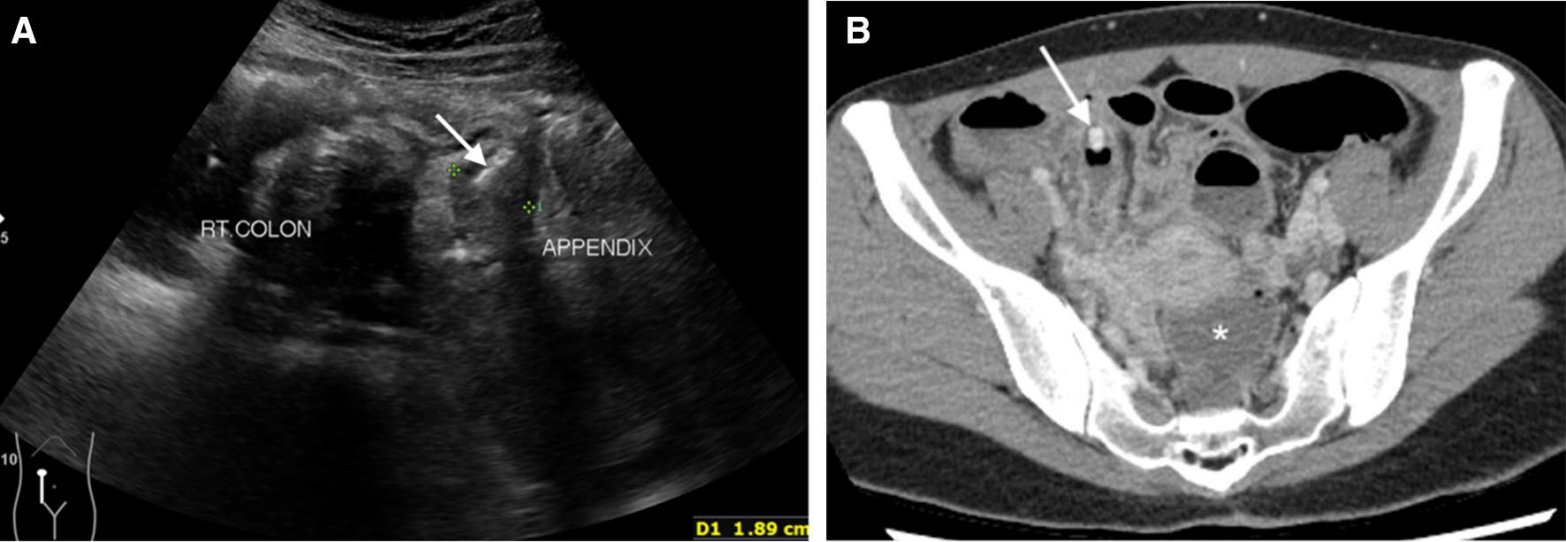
Intraluminal appendicolith. An ultrasound image a of a 35-year-old woman presenting with right lower abdominal pain for 1 day, elevated white blood cell counts (23,790 cells/mm^3^) and neutrophilia (93.3% neutrophils) shows a dilated appendix (arrows) with an intraluminal hyperechoic focus representing appendicolith. An obstructive appendicolith with acute appendicitis is confirmed on subsequent CT b that also reveals fluid in the cul-de-sac (asterisk) and peritoneal enhancement. Perforated appendicitis with turbid intraperitoneal fluid was confirmed at surgery
